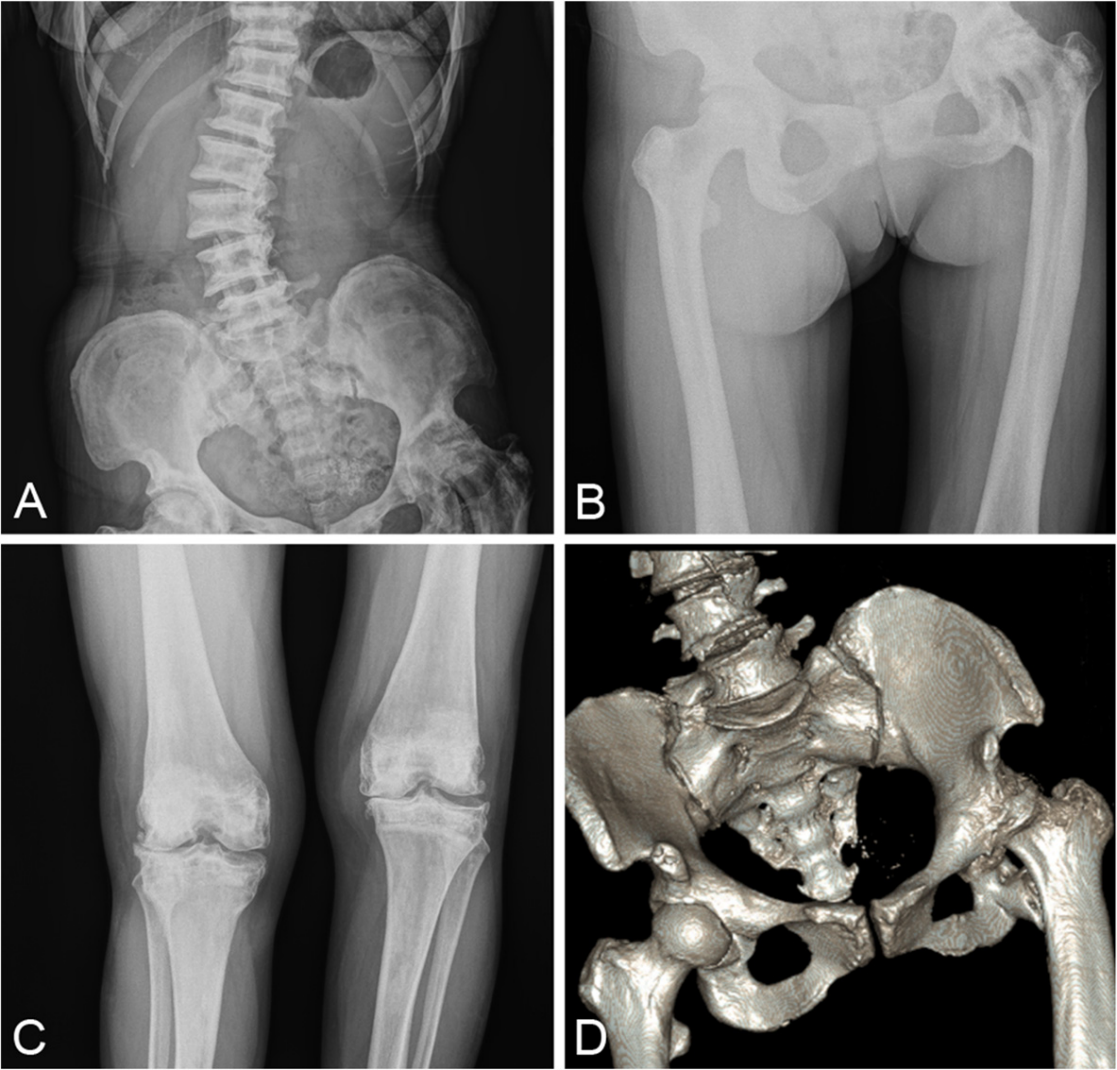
Preoperative radiographs of the spine, pelvic and knee. a, b, d X ray of pelvic showed endobones, and x ray of spine showed “rugger jersey spine”. The x-rays also showed severe osteoarthritis in the left hip with significant acetabular erosion, proximal migration of the femoral head and reduced joint space. c X-rays of the right knee showed similar osteoarthritic changed
